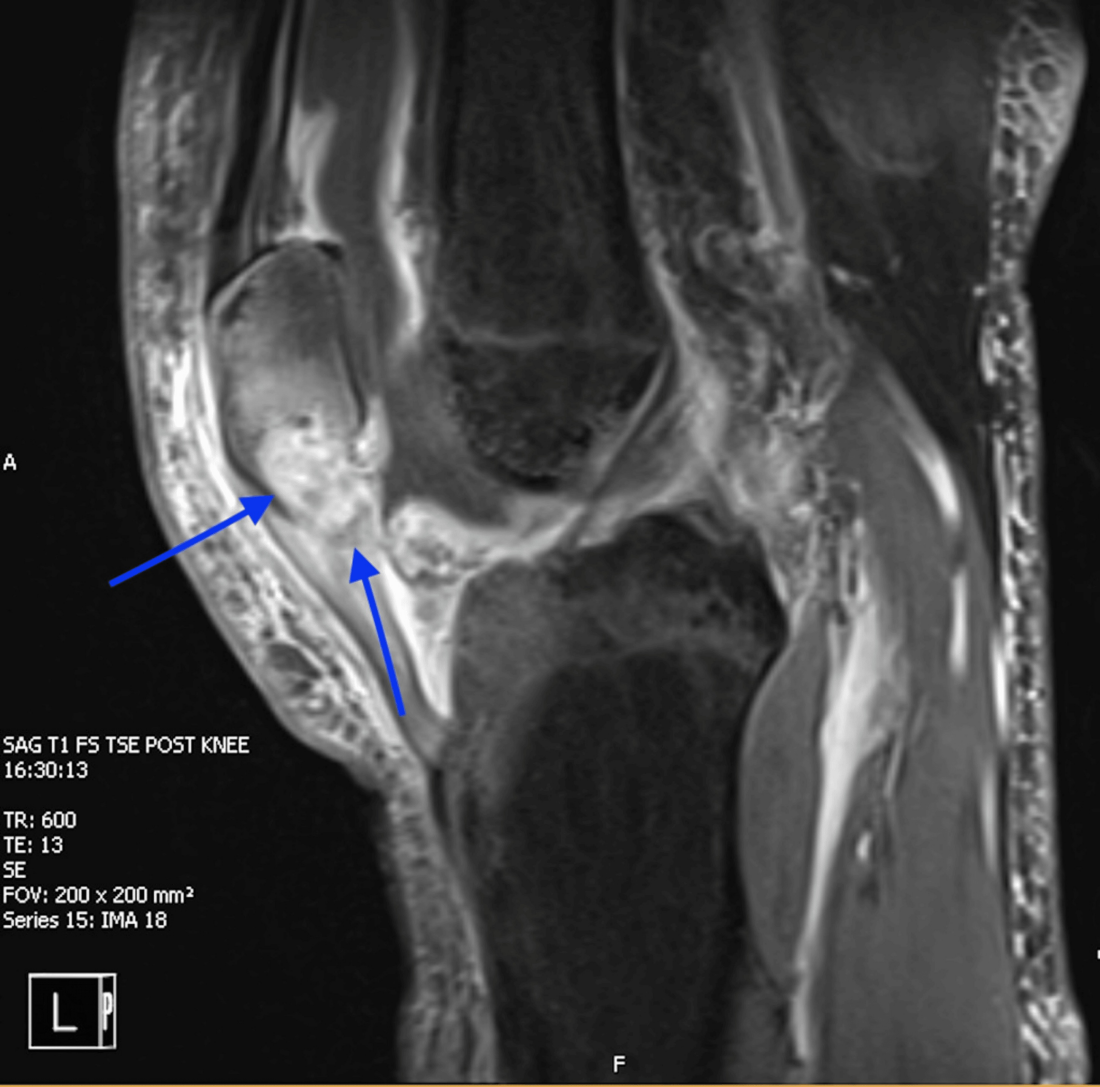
MRI of the knee post-contrast fat suppression T1 sequence showing a sagittal section demonstrating patellar osteomyelitis with synovial thickening
